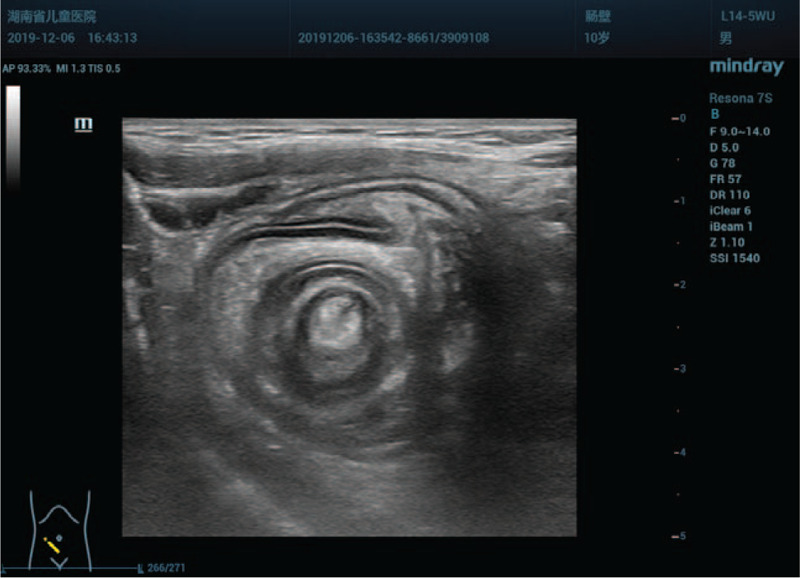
Axial sonogram of the right lower abdominal quadrant shows a target sign, the inner ring with a prominent central hyperecho representing the inverted appendix and mesoappendix.
